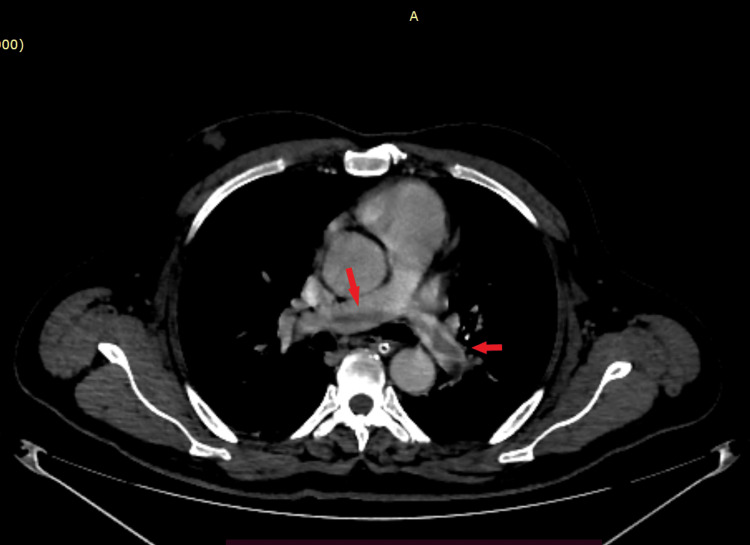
Contrast-enhanced CT thorax The red arrows indicate a saddle-shaped thrombus in the pulmonary trunk extending into the right and left pulmonary arteries.
